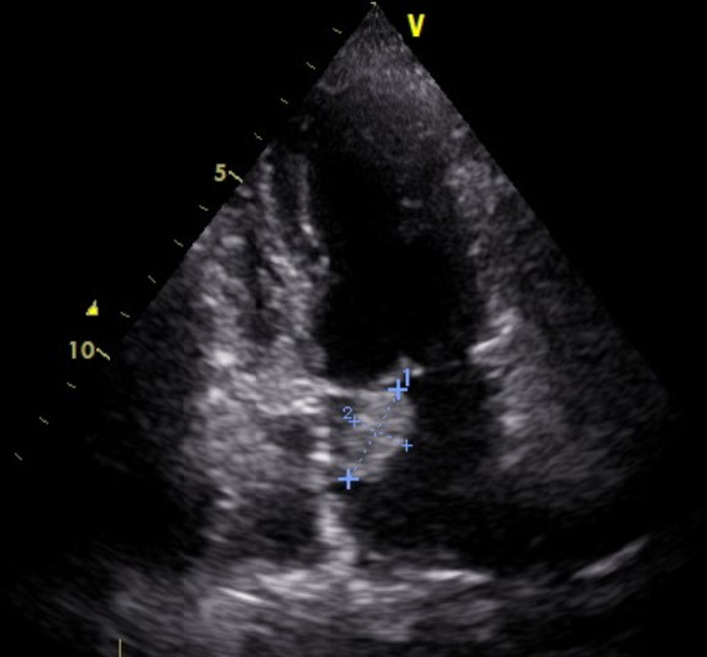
left atrial mass on transthoracic echocardiography (apical view)
